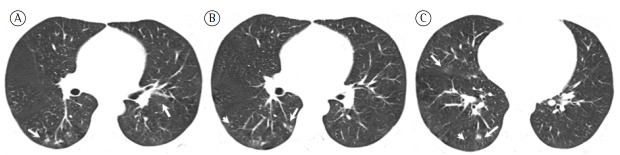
Chest CT scans at admission revealing hazy parenchymal micronodules
